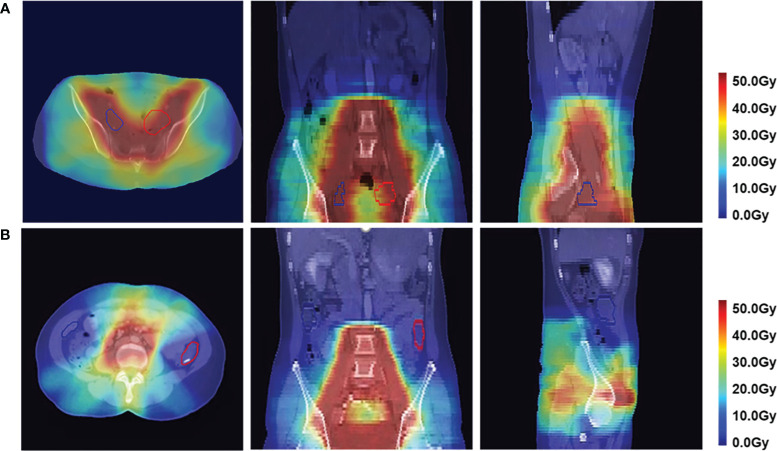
Dose distribution in axial, coronal, and sagittal views in color wash in a patient. The red contour is the left ovary, and the blue contour is the right ovary. (A) Predicted postoperative dose distribution overlaid on preoperative CT. (B) Postoperative dose distribution.
